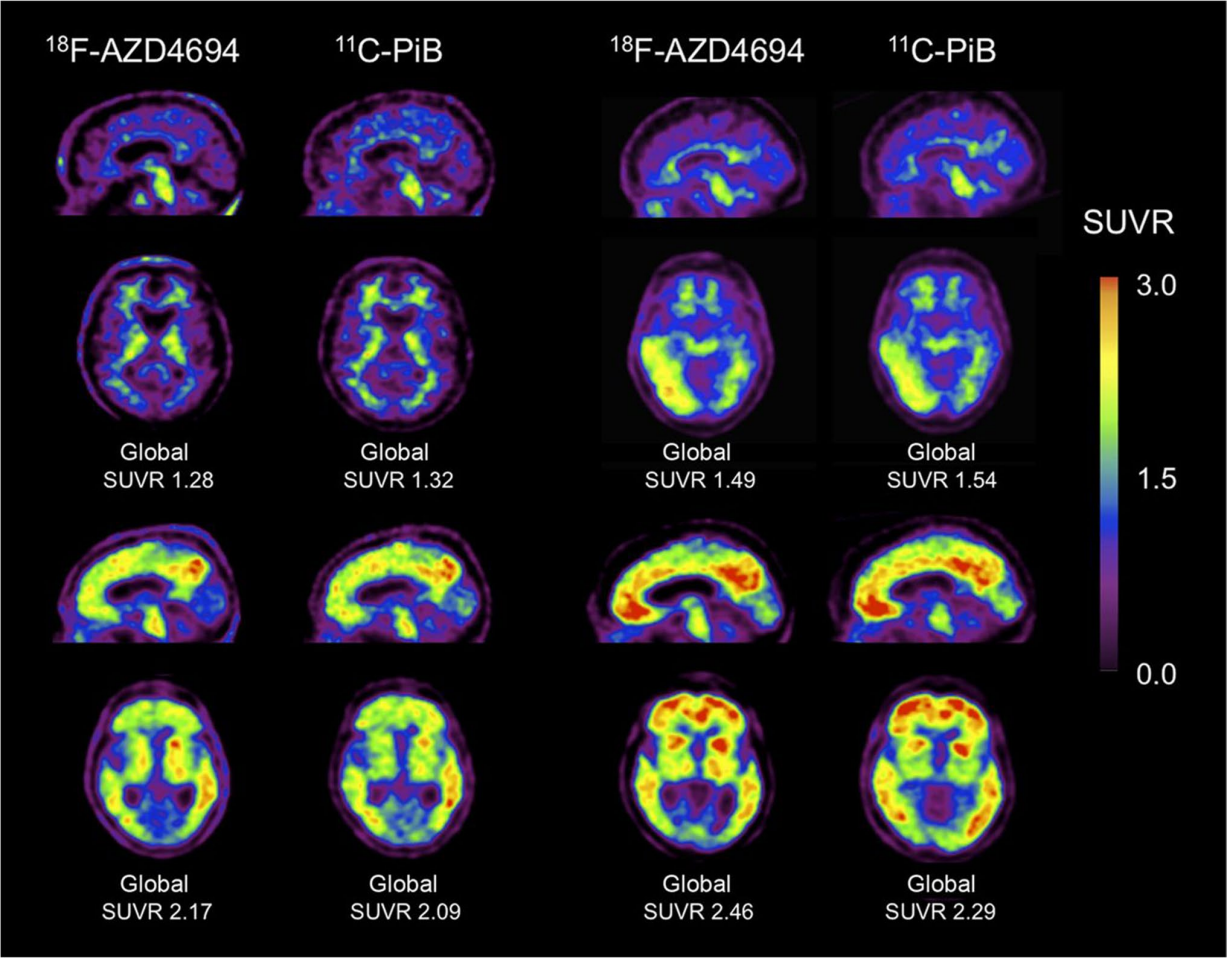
^18^F-AZD4694 and ^11^C-PiB PET imaging in 4 subjects. Four examples of sagittal and transaxial ^18^F-AZD4694 images adjacent to same-slice ^11^C-PiB images in same subject. Top 2 subjects were healthy age-matched controls, and bottom 2 were AD patients. The images showed near-identical appearance and dynamic range of SUVRs of ^18^F-AZD4694 and.^11^C-PiB. (This research was originally published in Journal of nuclear medicine: official publication, Society of Nuclear Medicine. 2013;54:880–6. Reprint permission was obtained)
